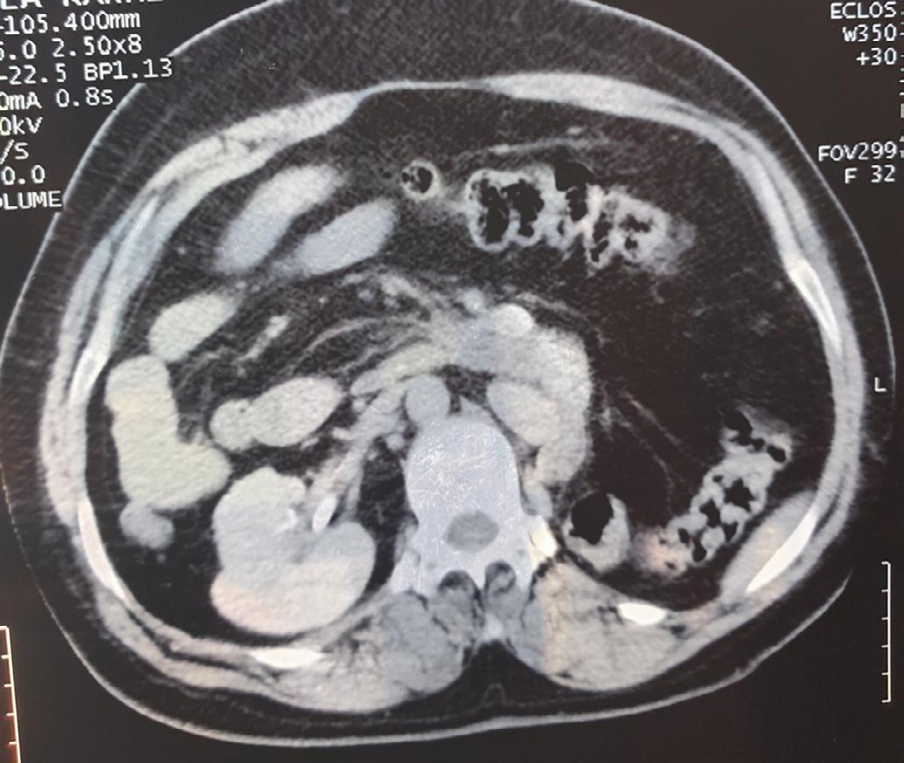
CT abdomen, left kidney cannot be visualized.
